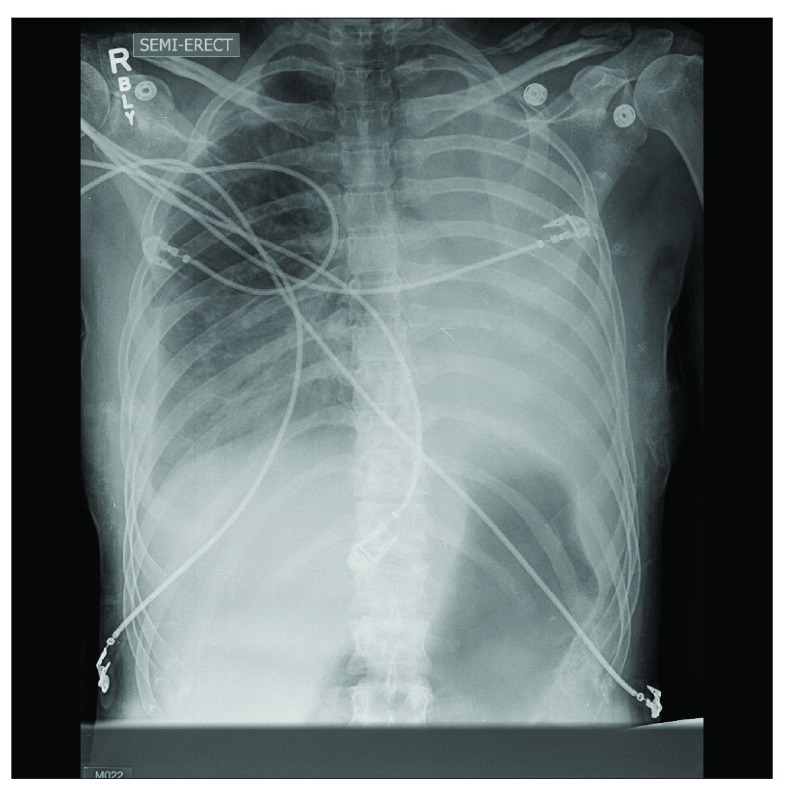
A preoperative chest X-ray showing complete collapse of the left lung.
